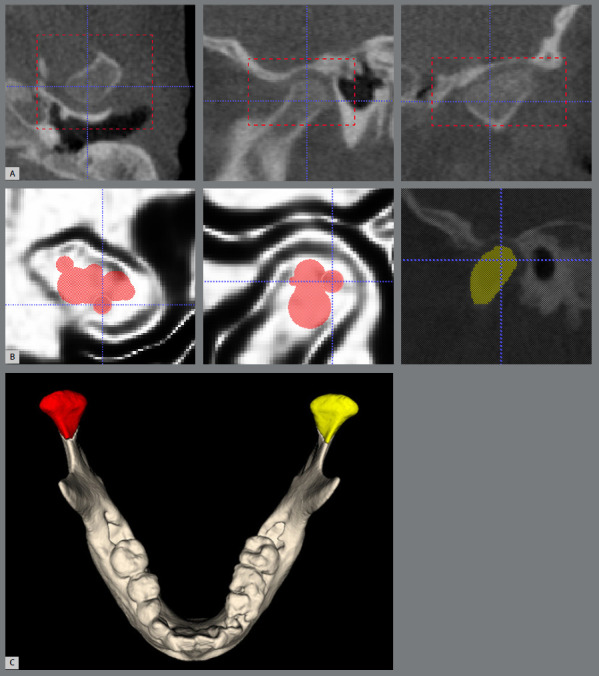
CBCT scans imported in ITK-SNAP software. A) Region of interest ( ROI ) of mandibular condyles in multiplanar reconstructions in the ITK-SNAP software. B) Application of bubble in “edge attraction” mode, for semi-automatic segmentation, and the segmented condyle in yellow. C) 3D virtual model after segmentation.
